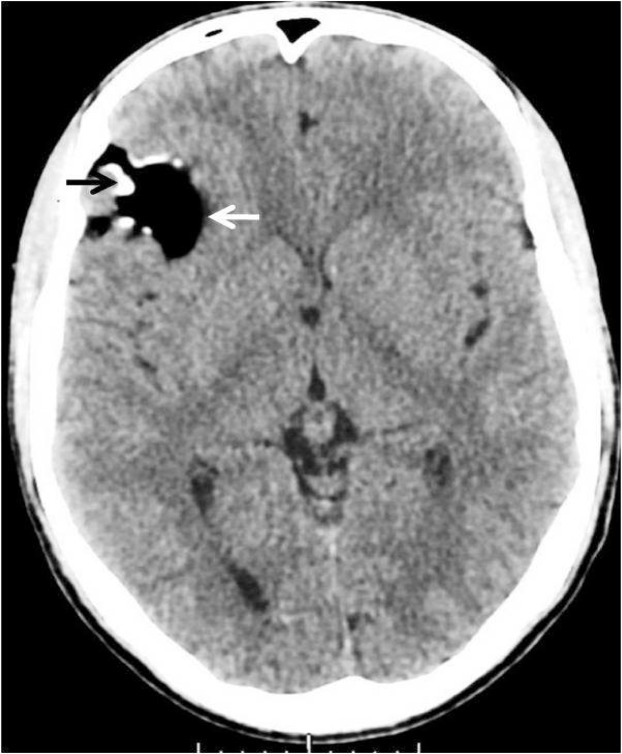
Unenhanced axial CT image shows a large homogeneous right sylvian fissure lipoma with CT value of fat (white arrow). Thick linear calcification is seen at its periphery (black arrow).
